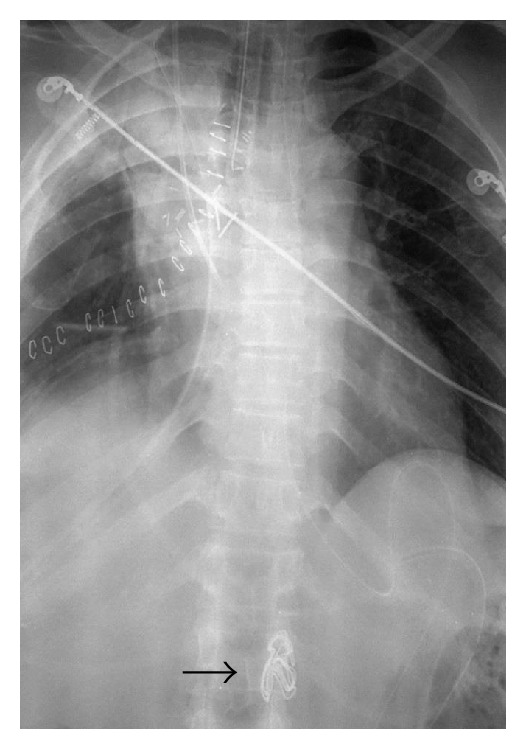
X-ray of chest showing location of the surgical swab (arrow).
